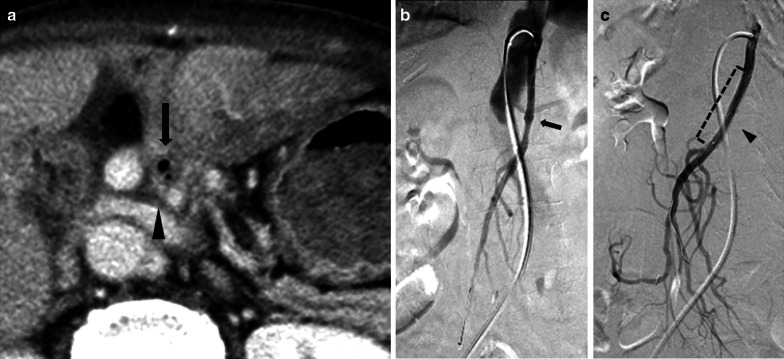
Evaluation using axial equilibrium phase contrast-enhanced computed tomography (CECT). a Axial equilibrium phase CECT shows the collection of gas (black arrow), an aneurysm of the superior mesenteric artery (black arrowhead) and the stomach filling with contrast fluid. b Superior mesenteric arteriography shows an aneurysm (black arrow), but extravasation was uncertain. c Arteriography after endovascular repair shows resolution of the aneurysm and preserved SMA blood flow through the covered stent (black arrowhead and dotted line)
